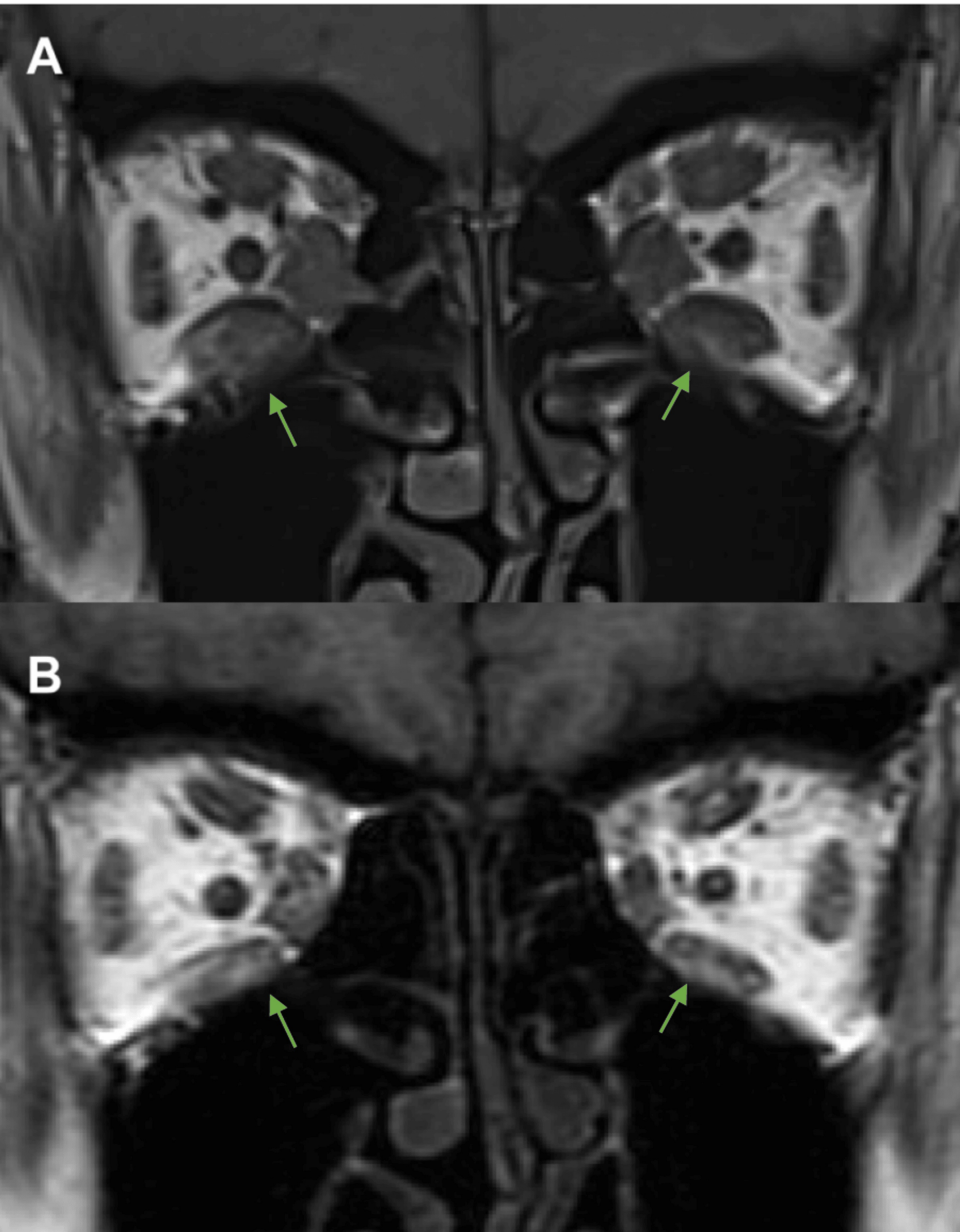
MRI findings (T1-weighted images) of Graves’ orbitopathy with dysthyroid optic neuropathy before (A) and eight weeks after (B) sheath-guided orbital triamcinolone injections. Arrows indicate the decrease in size of the more prominent bilateral inferior rectus muscles.
